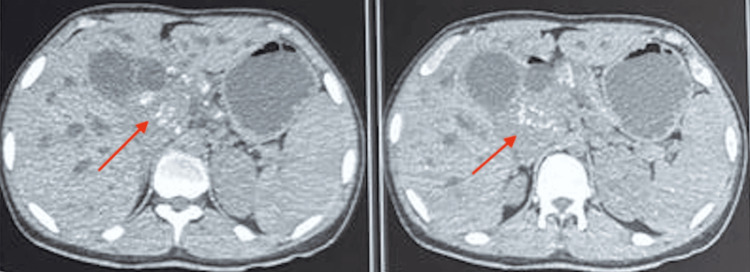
Contrast-enhanced CT (CECT) abdomen showing a periportal lymph nodal mass Axial CECT images of the abdomen demonstrate a heterogeneous, enhancing soft-tissue mass in the periportal region (red arrows). The lesion is seen encasing and compressing the common bile duct, resulting in upstream biliary dilatation.
